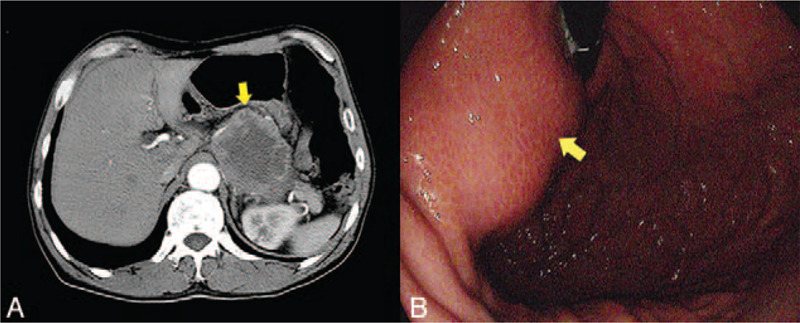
Images of CT and gastroscopy. Contrast-enhanced CT showed a large exophytic mass (yellow arrow) on the body of the stomach (A). The gastroscopy indicated a submucosal mass (yellow arrow) (B).
